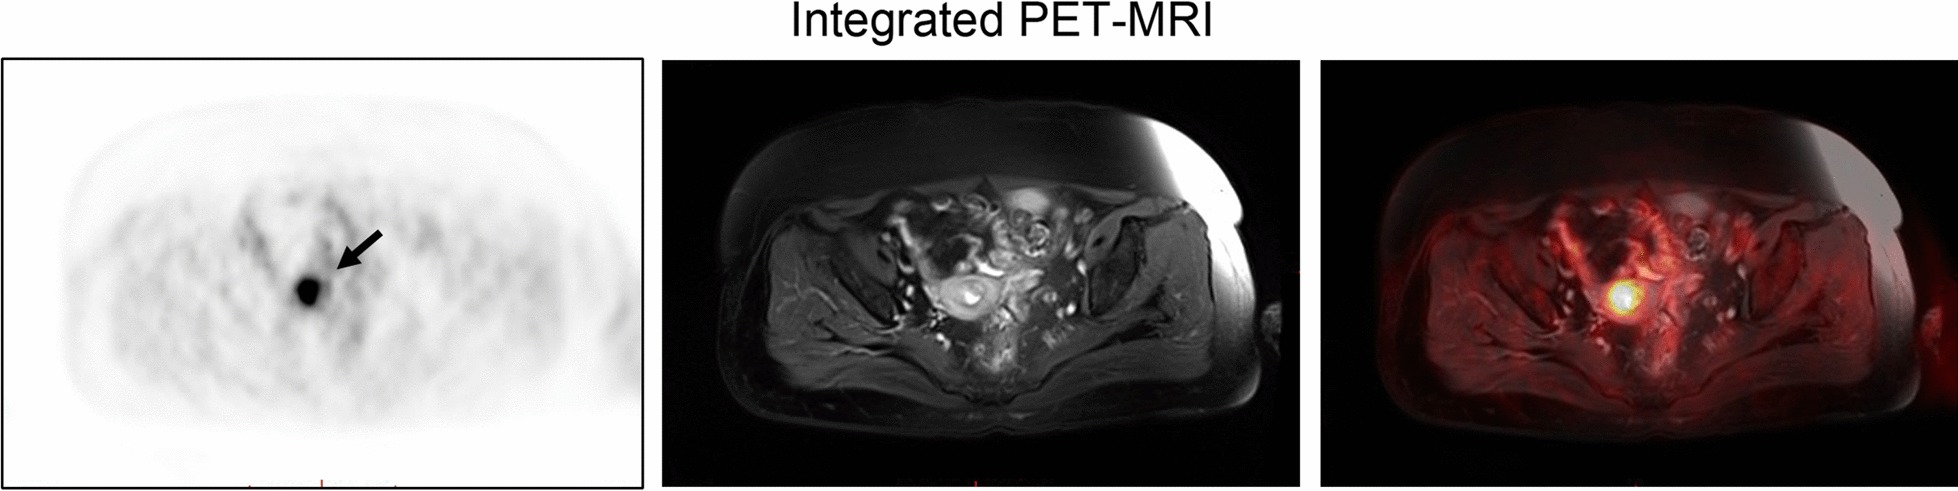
One patient with endometrial cancer. Axial integrated PET/MRI shows intense ^18^F-FDG uptake by uterine cavity (black arrow).
